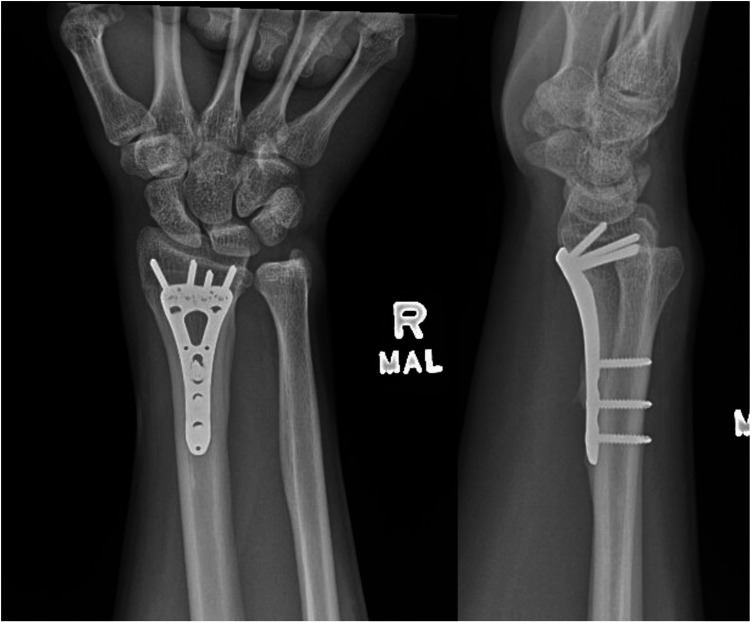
Postoperative radiographs. Radiographs done postoperative day 141 demonstrate stable alignment of the fracture and hardware.
